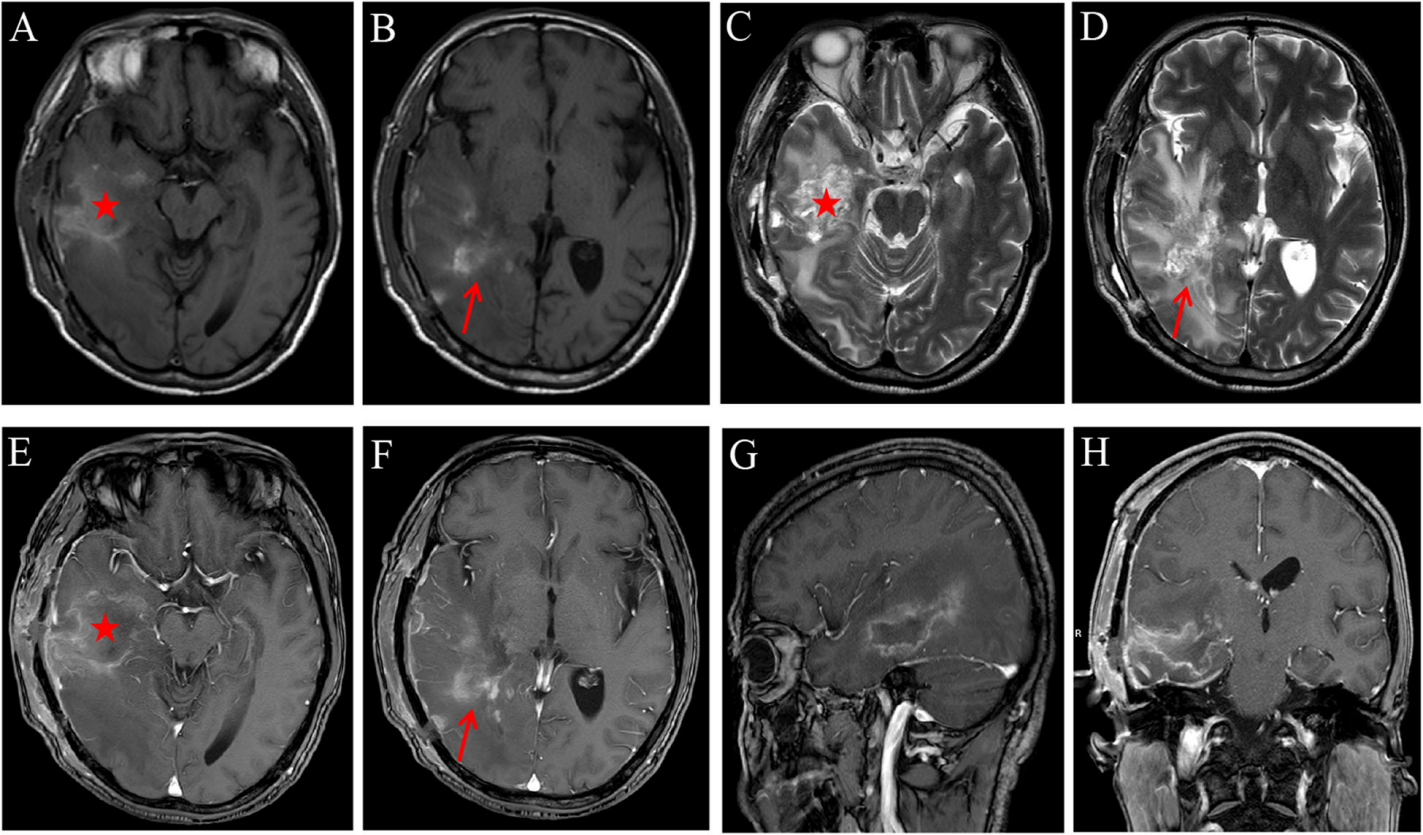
Head MRI images after the second surgery. a-d Plaque-like long T1-weighted and T2-weighted signal shadows in the right temporal lobe and occipital lobe. e-h No substantially enhanced signal after enhancement. Asterisk = the area of the abscess in the first admission. Arrow = the area of the recurrent abscess
